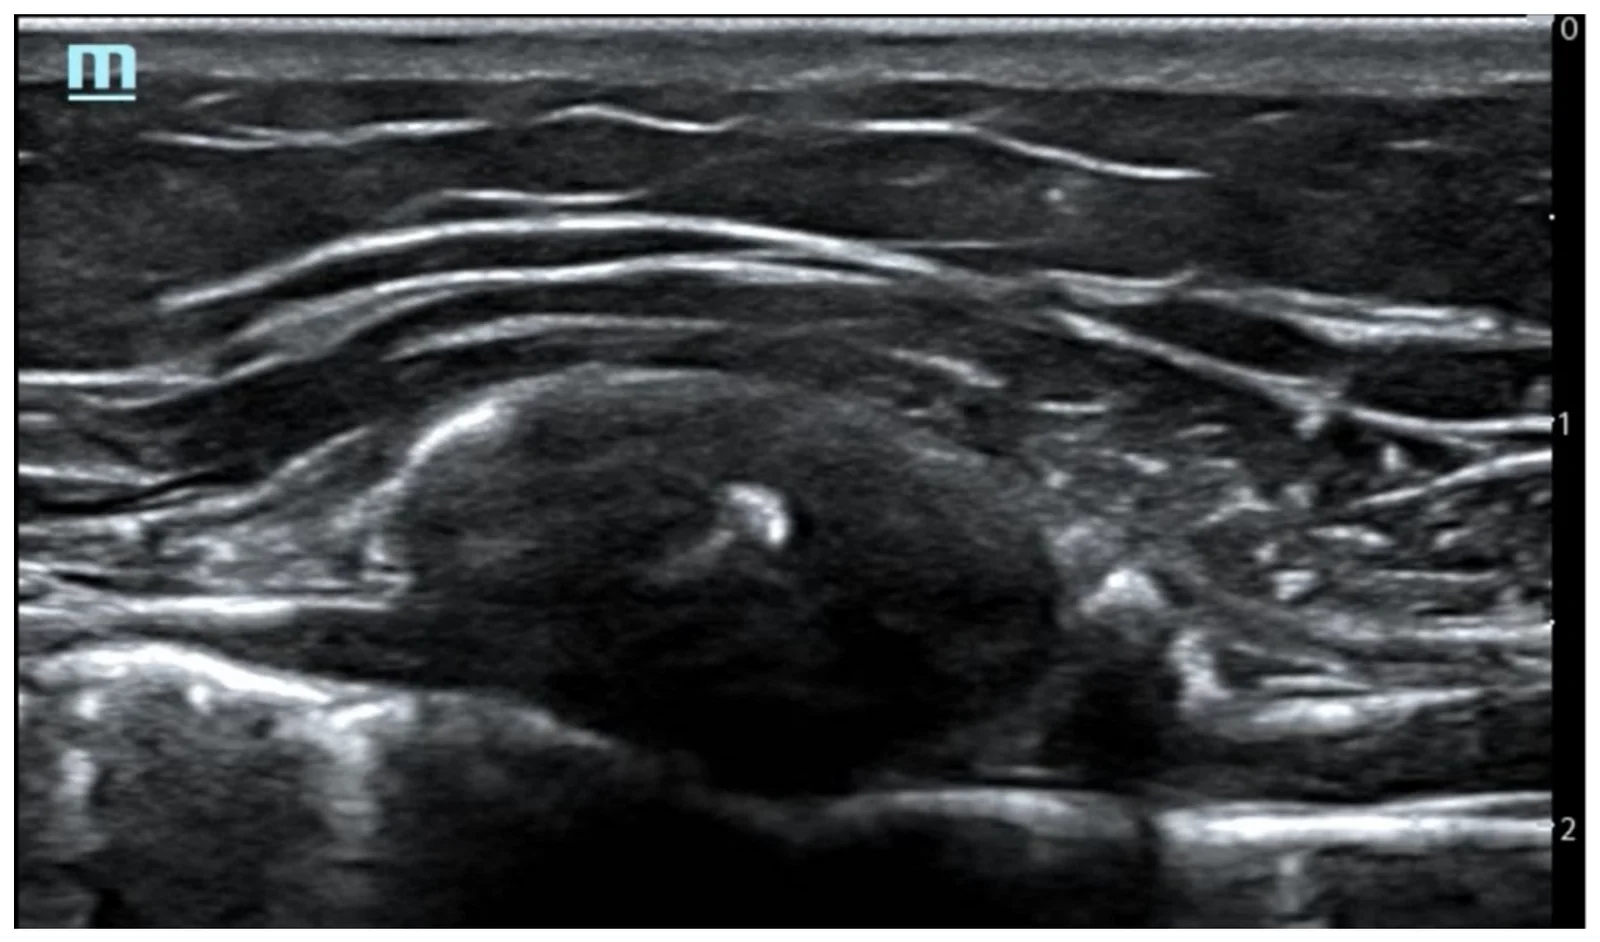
Ultrasound view of pectoralis major muscle resting on rib cartilage in parasternal line.
